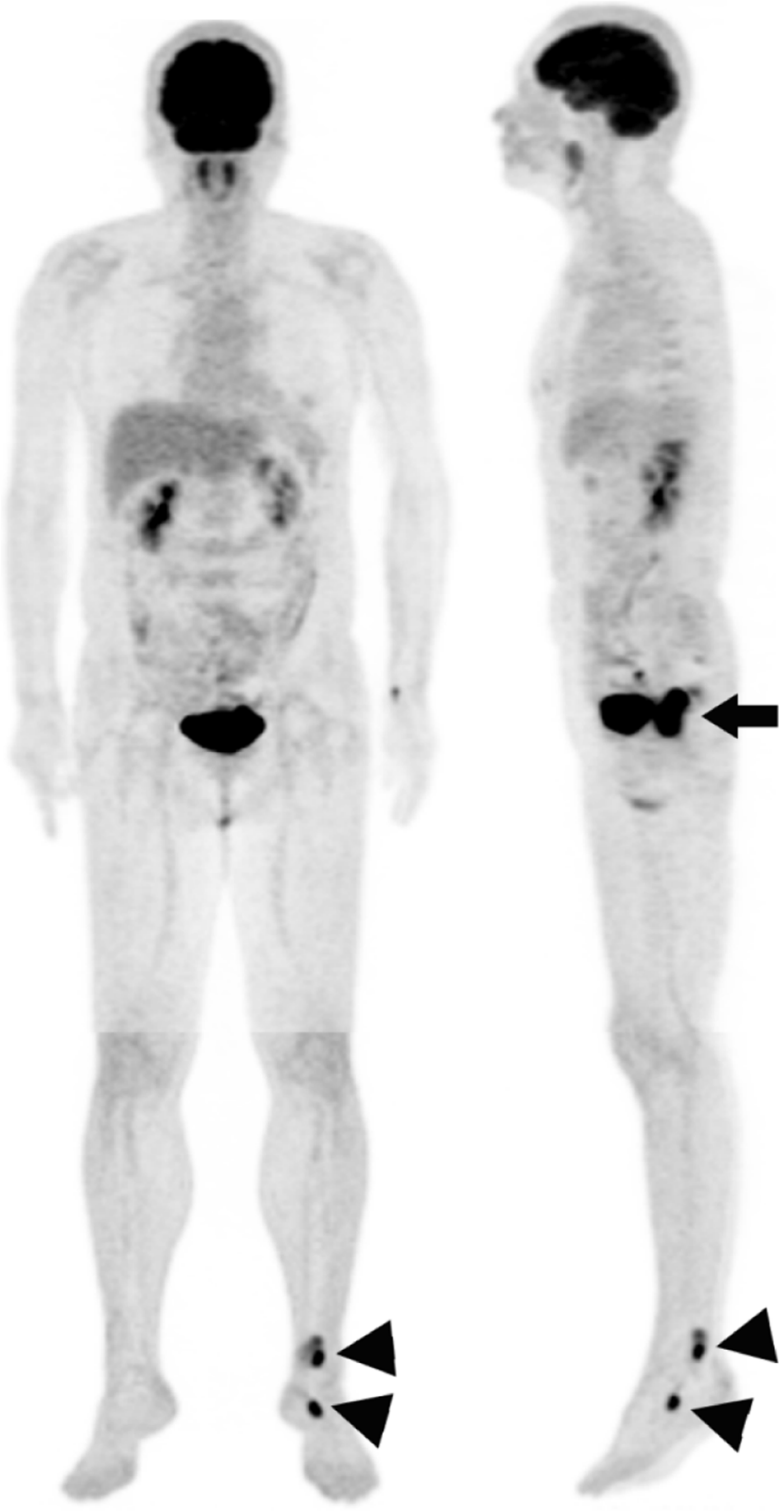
Anterior (left) and left lateral (right) whole-body Fluorine-18 fluorodeoxyglucose (^18^F-FDG) maximum-intensity projections in a patient with rectal melanoma. An intensely ^18^F-FDG-avid lesion was present in the rectum (arrow) and two additional intensely ^18^F-FDG-avid lesions were present in the left ankle and in the left foot (arrowheads)
